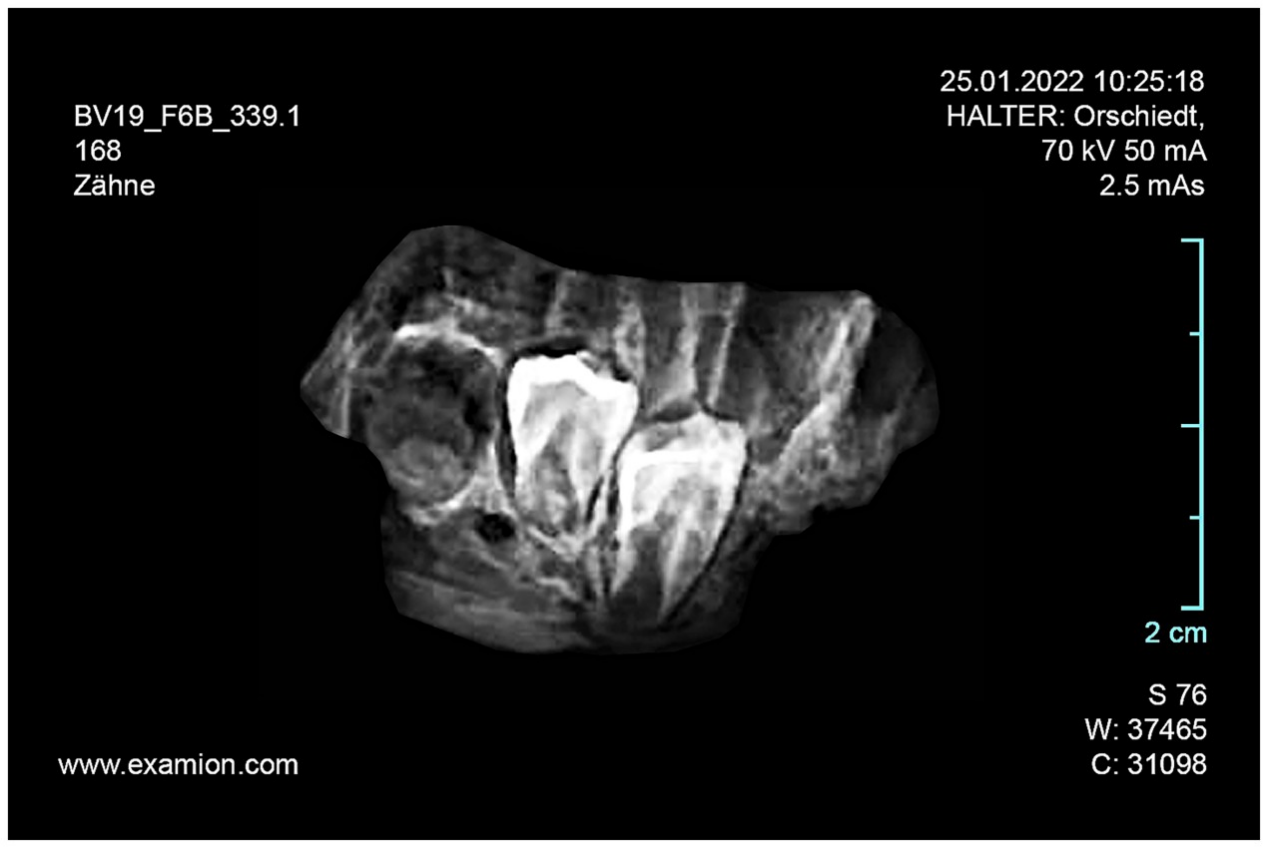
X-ray of the mandibular fragment showing the crowns of not yet erupted permanent teeth. –Photo: J. Orschiedt, LWL-AfW Olpe/A. Müller.
